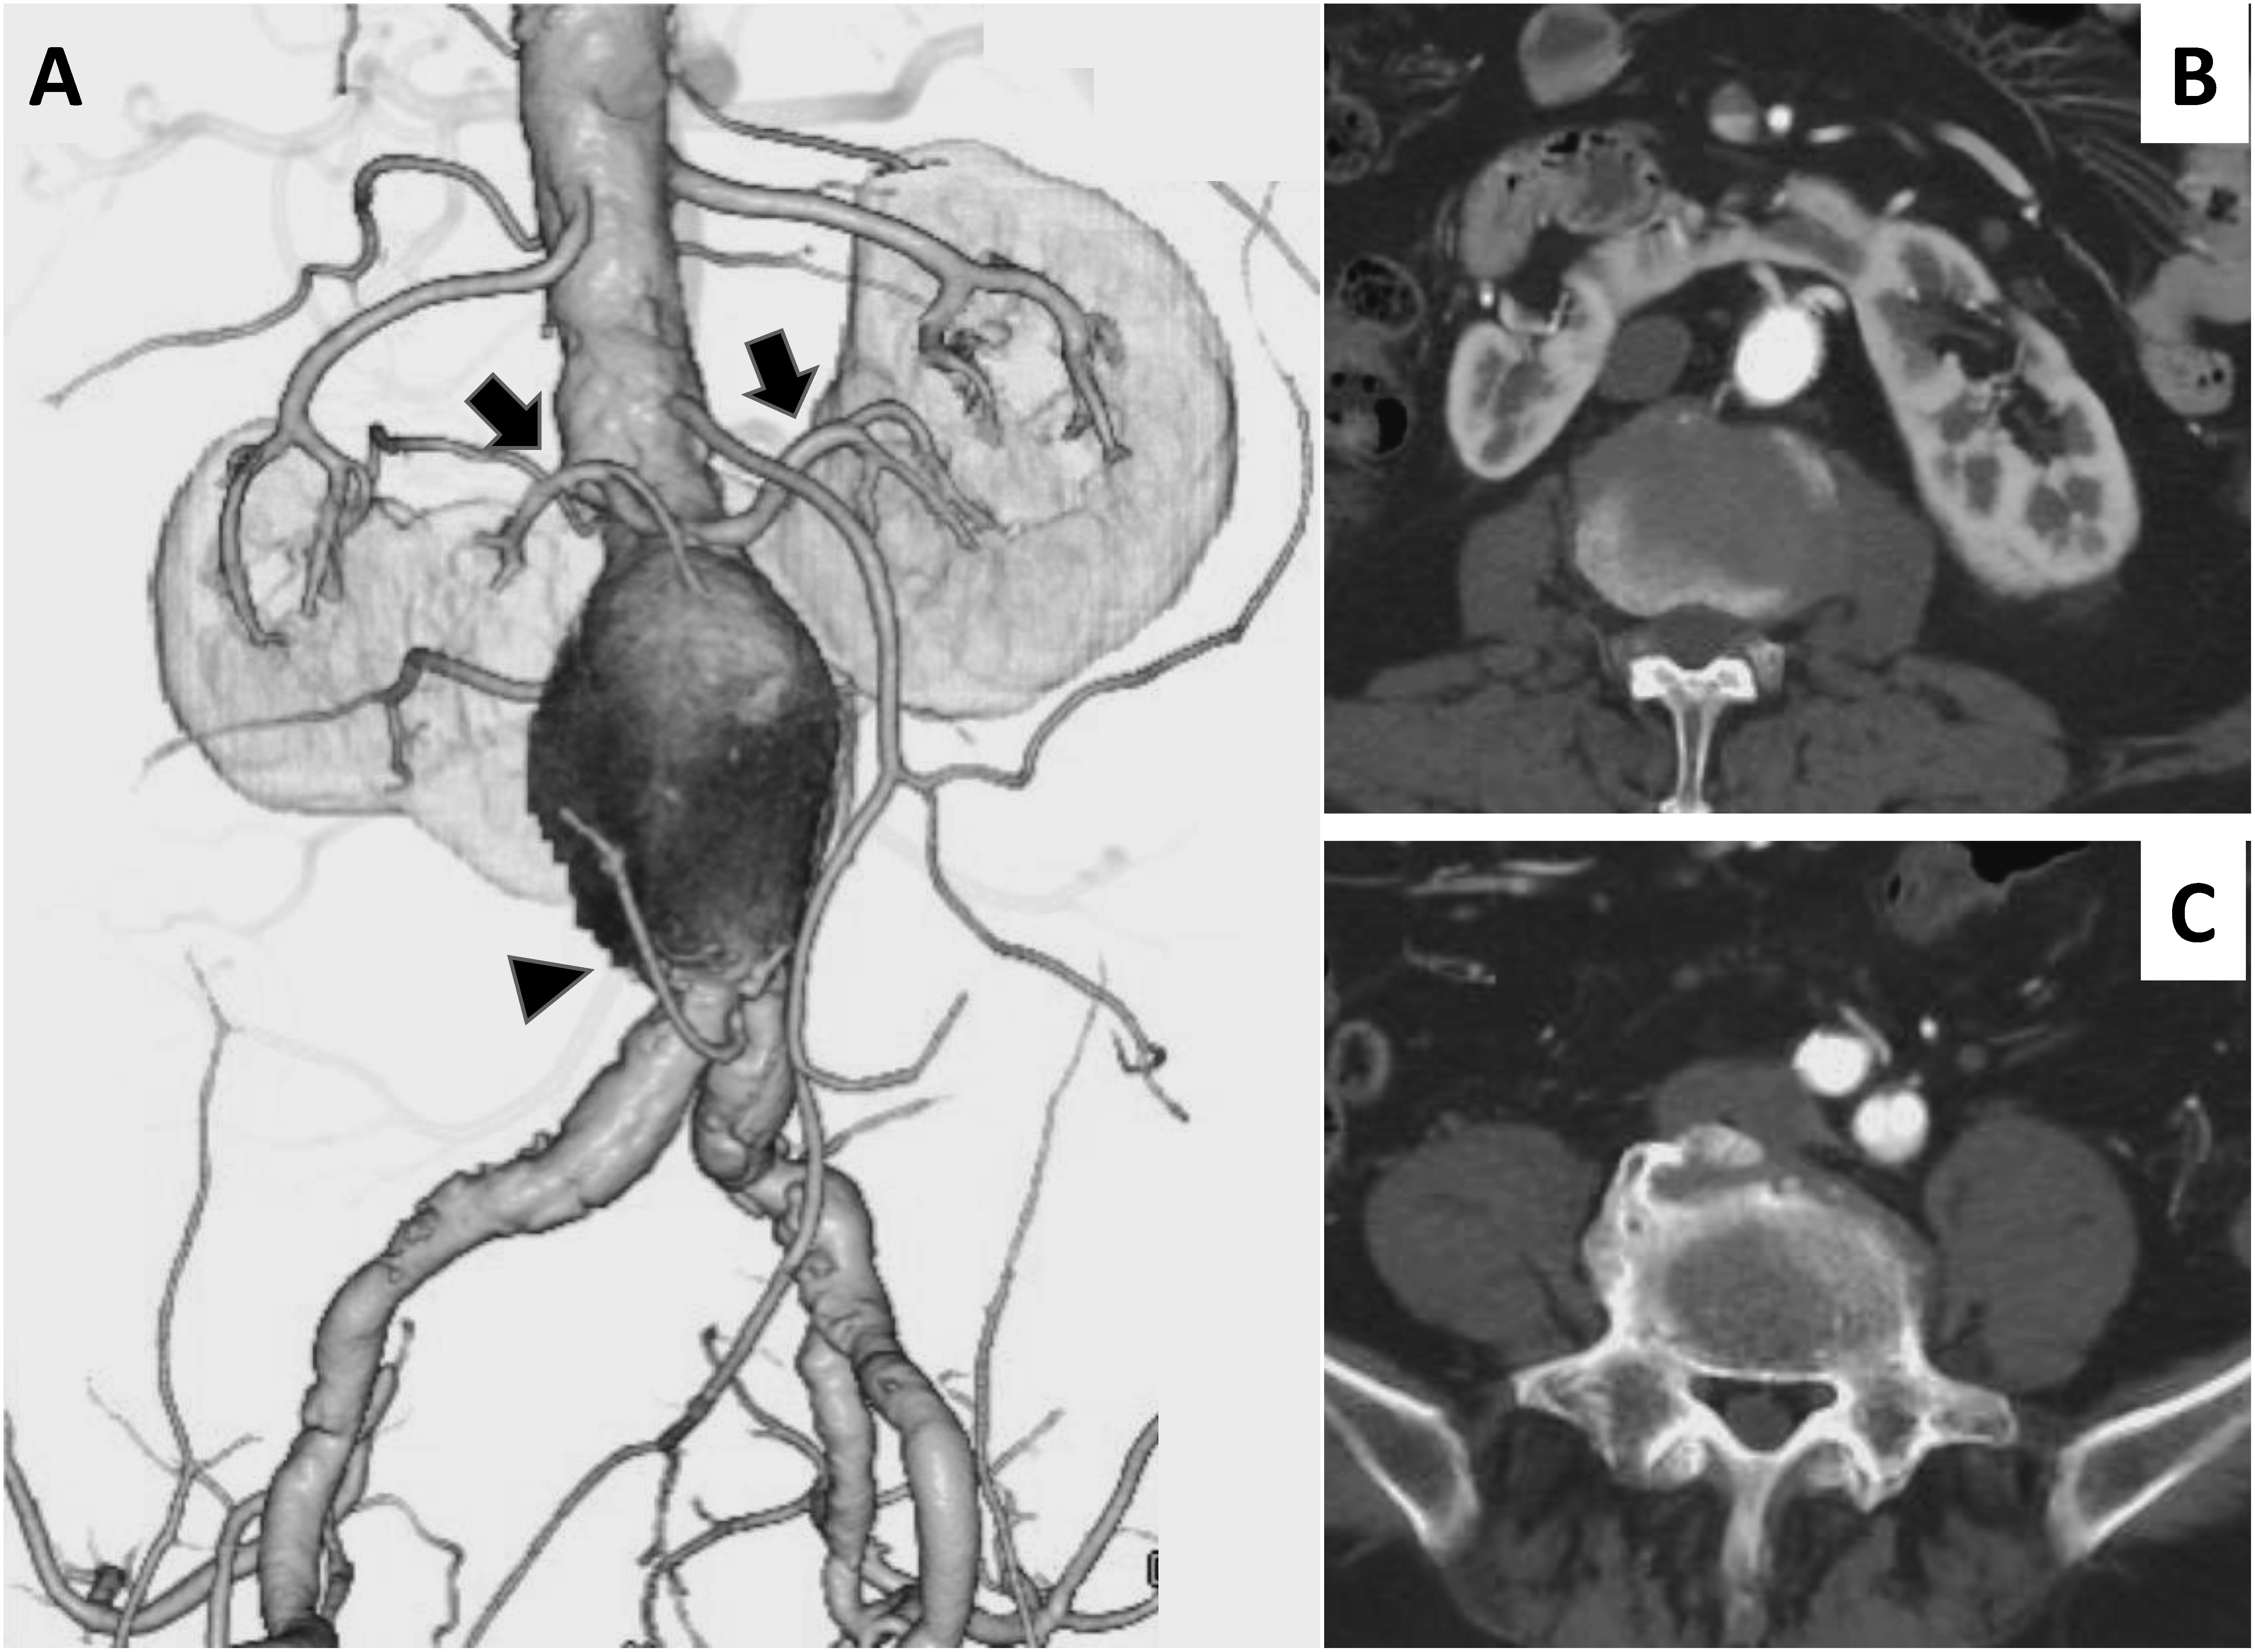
Fig. 1 Preoperative contrast-enhanced computed tomography (CT).
